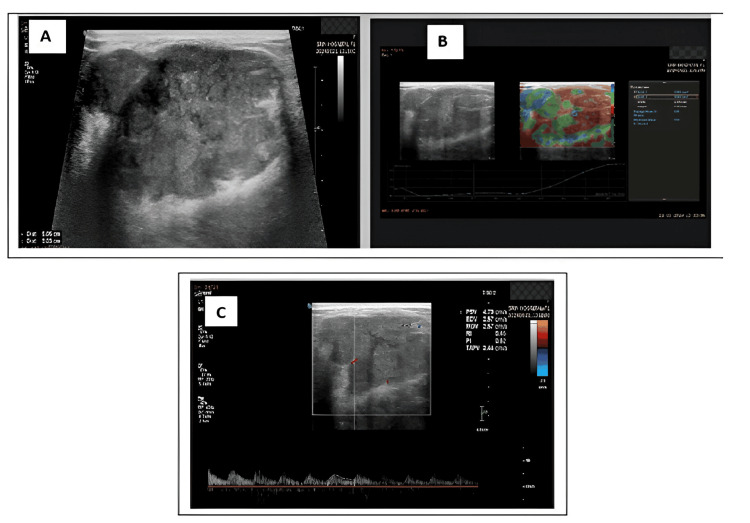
A 43-year-old female patient presented with right breast pain A: Greyscale ultrasound revealed a well-defined, wider-than-taller, macro-lobulated lesion with lobulated margins in the right upper outer quadrant and classified it as BI-RADS 3. B: Strain elastography showed a strain ratio of 2.29. C: Doppler revealed a PI of 0.62 and a RI of 0.45. However, FNAC confirmed the lesion as mucinous carcinoma. PI: pulsatility index; RI: resistance index; FNAC: fine needle aspiration cytology.
